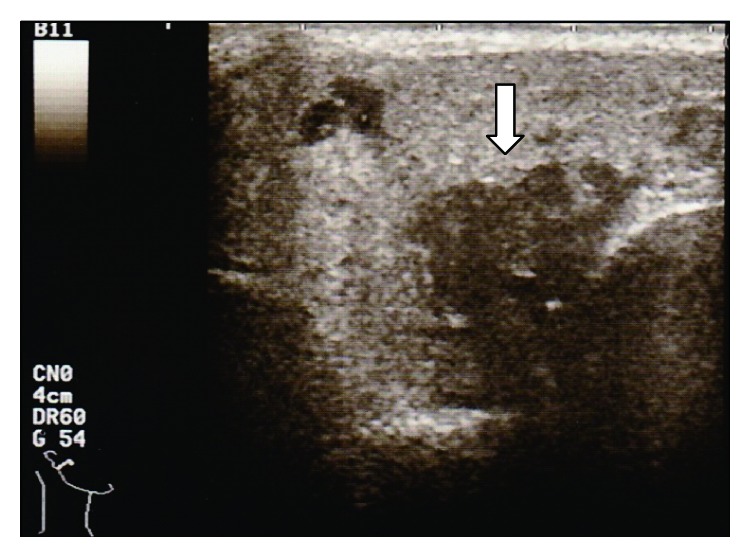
Ultrasound image for Case  1, showing the enlarged right parotid gland and interspersed hypoechoic areas (arrow).
